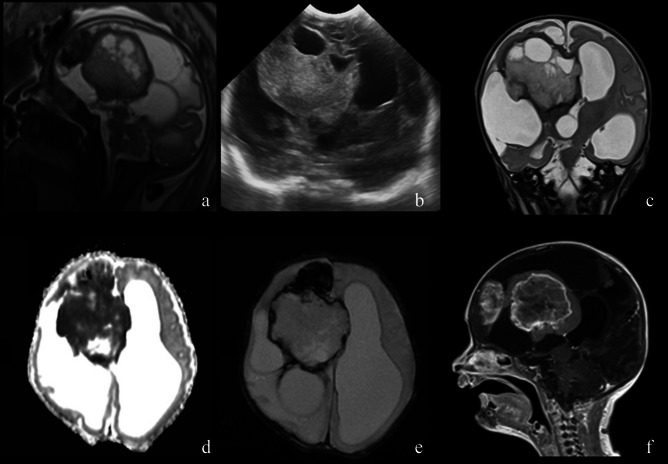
Congenital Infant-Type Hemispheric Glioma* in a newborn. Foetal US at 35 weeks (sagittal plane, a), and neonatal US (coronal plane, b) shows a large solid-cystic and haemorrhagic mass in the right lateral ventricle. MRI images (c–f) confirm the solid-cystic hypercellular mass with blood products and heterogeneous enhancement that obliterates the right foramen of Monro causing compression and contralateral displacement of the third ventricle and severe hydrocephalus. Molecular analysis revealed NTRK1 Fusion
